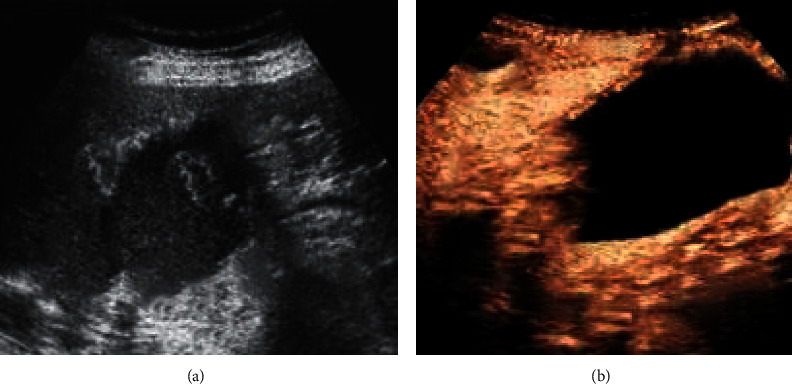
Contrast ultrasound image: (a) two-dimensional ultrasound showing thickening of the gallbladder wall, multiple stones, and linear echo in the cyst cavity, which was considered the intima shedding; (b) contrast ultrasound showing discontinuous enhancement of the gallbladder wall, with focal defects, and the falling intima within the cyst cavity was not reinforced.
